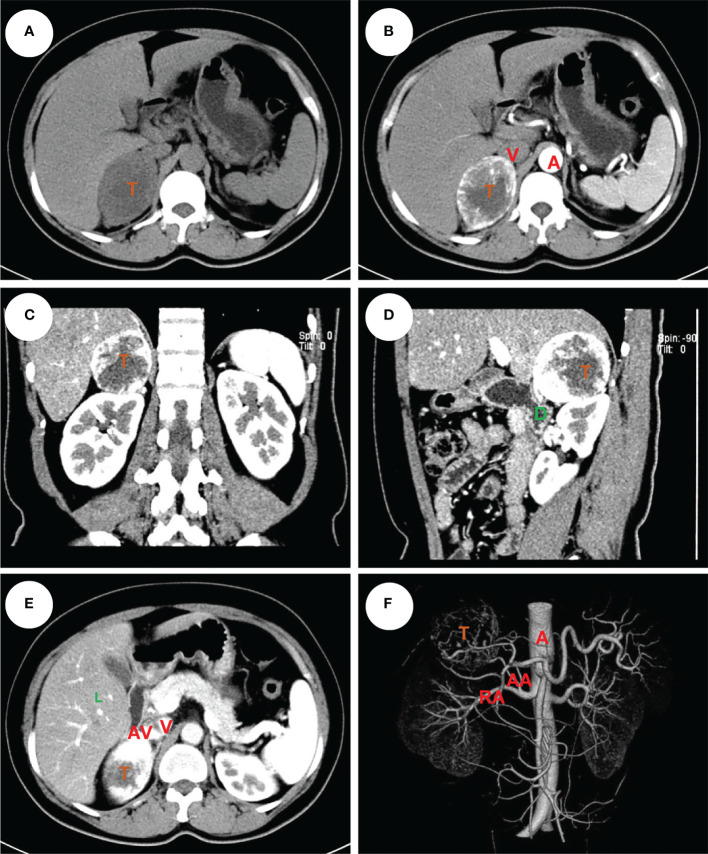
An example of right pheochromocytoma. The female patient had a 6 cm pheochromocytoma arising from the right adrenal gland (A) with 120 HU on contrast-enhanced CT (B). A clear tumor boundary was observed on transverse (B), coronal (C) and sagittal (D) section which revealed a close relationship between the tumor and ipsilateral kidney, right hepatic lobe and duodenum. An arterial-venous phase section (E) showed a short adrenal vein draining directly into the vena cava. The 3-D vessel reconstruction based on arterial phase CT scan demonstrated the relative location of the tumor to surrounding major abdominal vessels (F). The tumor was removed robotically *via* retroperitoneal approach. The patients had an ADRENAL score of 2_A_ + 1_D_ + 0_R_ + 2_E_ + 1_N_ + 1_A_ + R_L_ = 7R. T, tumor; L, liver; D, duodenum; A, aorta; V, (inferior) vena cava; RA, renal artery; AA, (middle) adrenal artery; AV, adrenal vein.
